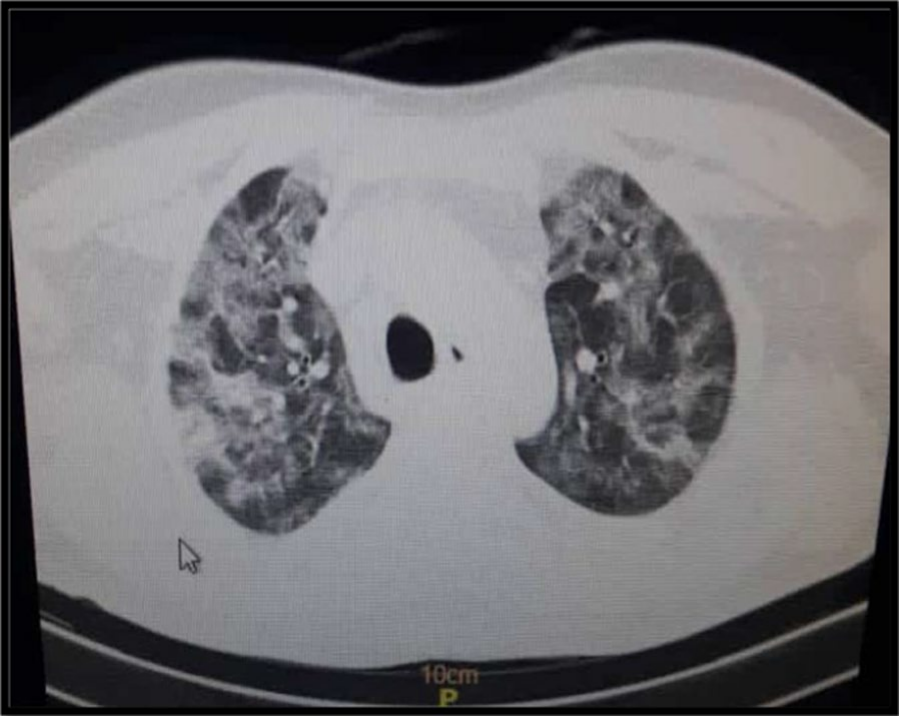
Computed tomography (CT) scan of the chest of a patient with COVID-19 shows multiple patchy ground-glass opacities
